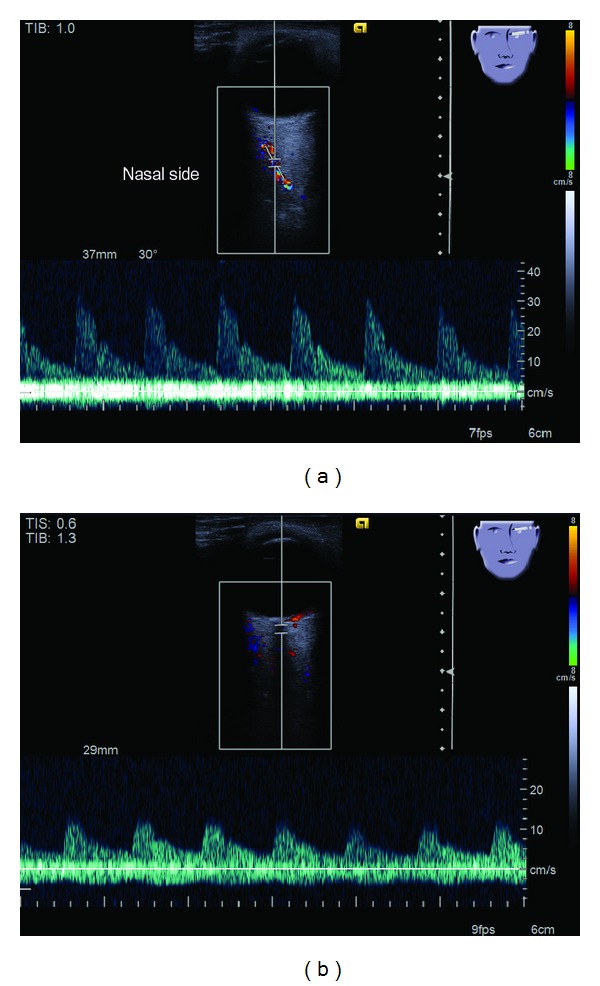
Color Doppler flow imaging of the ophthalmic artery (a) and central retinal artery (b) in normal control.
